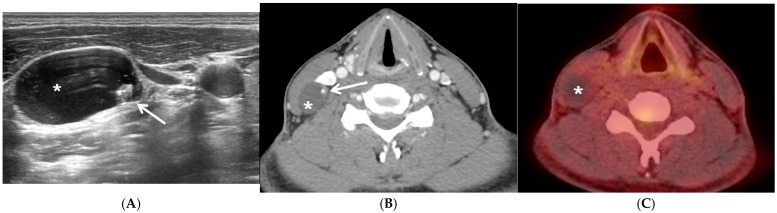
Representative case of papillary thyroid carcinoma in solitary lateral neck cystic mass without identifiable primary carcinoma within the thyroid. Preoperative ultrasonography (US) (A) and computed tomography (CT) (B) showing a 25 mm × 16 mm-sized lobular, well-defined, cystic mass (asterisk) containing a solid and hyperechoic lesion with punctate calcification (white arrow) in the right neck at level III (A,B) without other enlarged lymph nodes on either side of the neck and normal findings in the thyroid. The cystic lateral neck mass in the right neck exhibited no obvious F-18 fluorodeoxyglucose (FDG) uptake or any other suspicious F-18 FDG-avid lesions documented on positron emission tomography/CT (PET/CT) (C).
